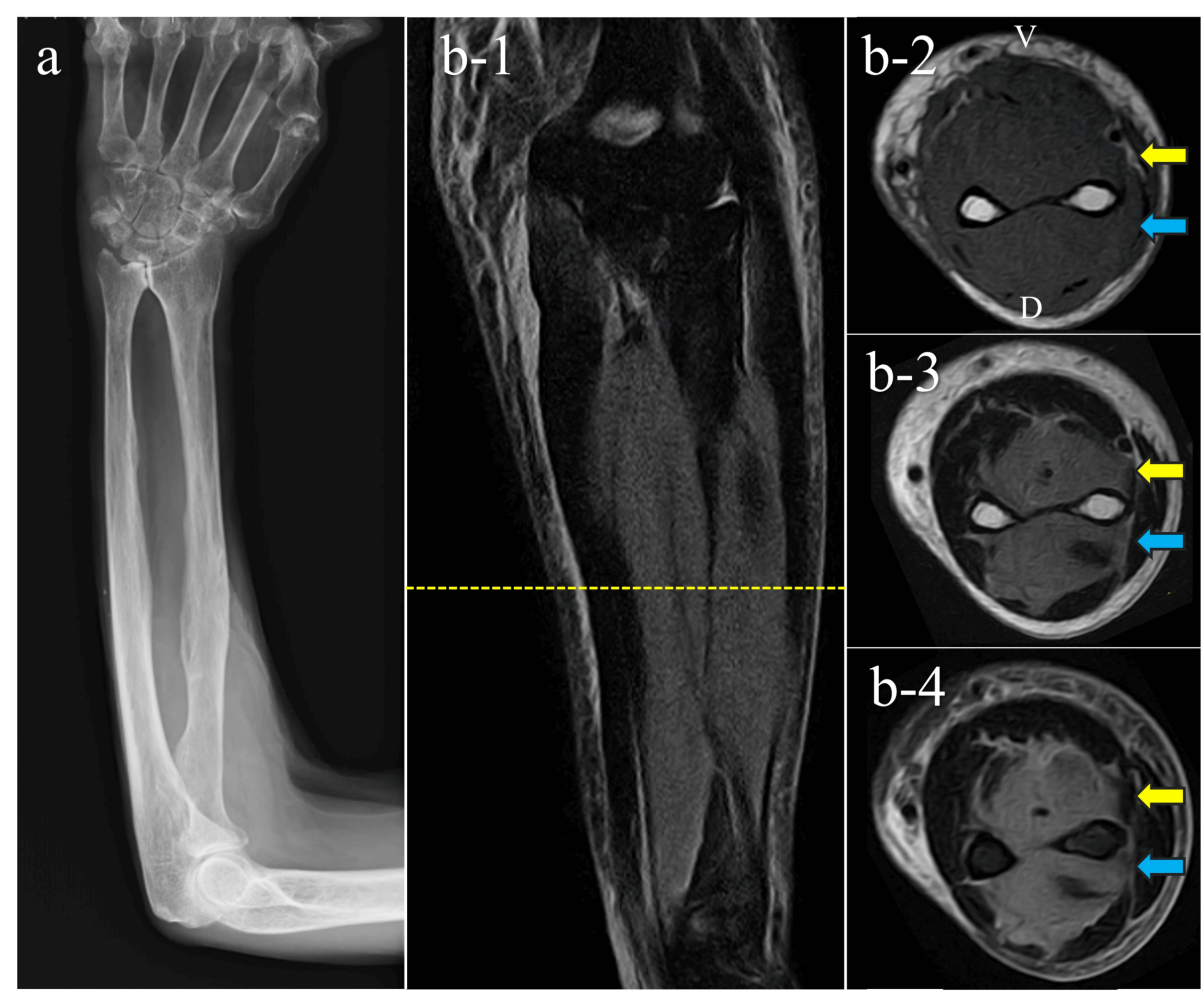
Imaging studies done at the second visit. (a) Plain radiograph of the forearm; (b) MRI of the forearm: (b-1) STIR coronal image, (b-2) T1-weighted axial image, (b-3) T2-weighted axial image, (b-4) STIR axial image. V, volar side; D, dorsal side; MRI, magnetic resonance imaging; STIR, short tau inversion recovery. Yellow dotted line indicates the slice level corresponding to images b-2 to b-4. Yellow arrows indicate the lesion in the deep volar compartment. Blue arrows indicate the lesion in the dorsal compartment.
